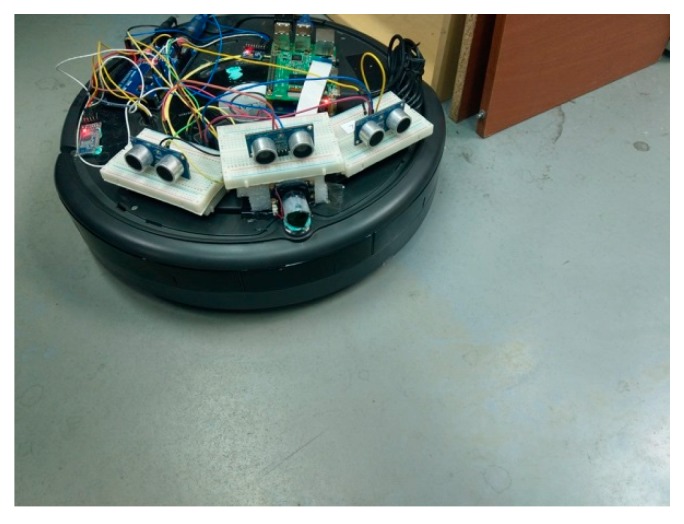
Three ultrasonic sensors installed on the clean robot.
